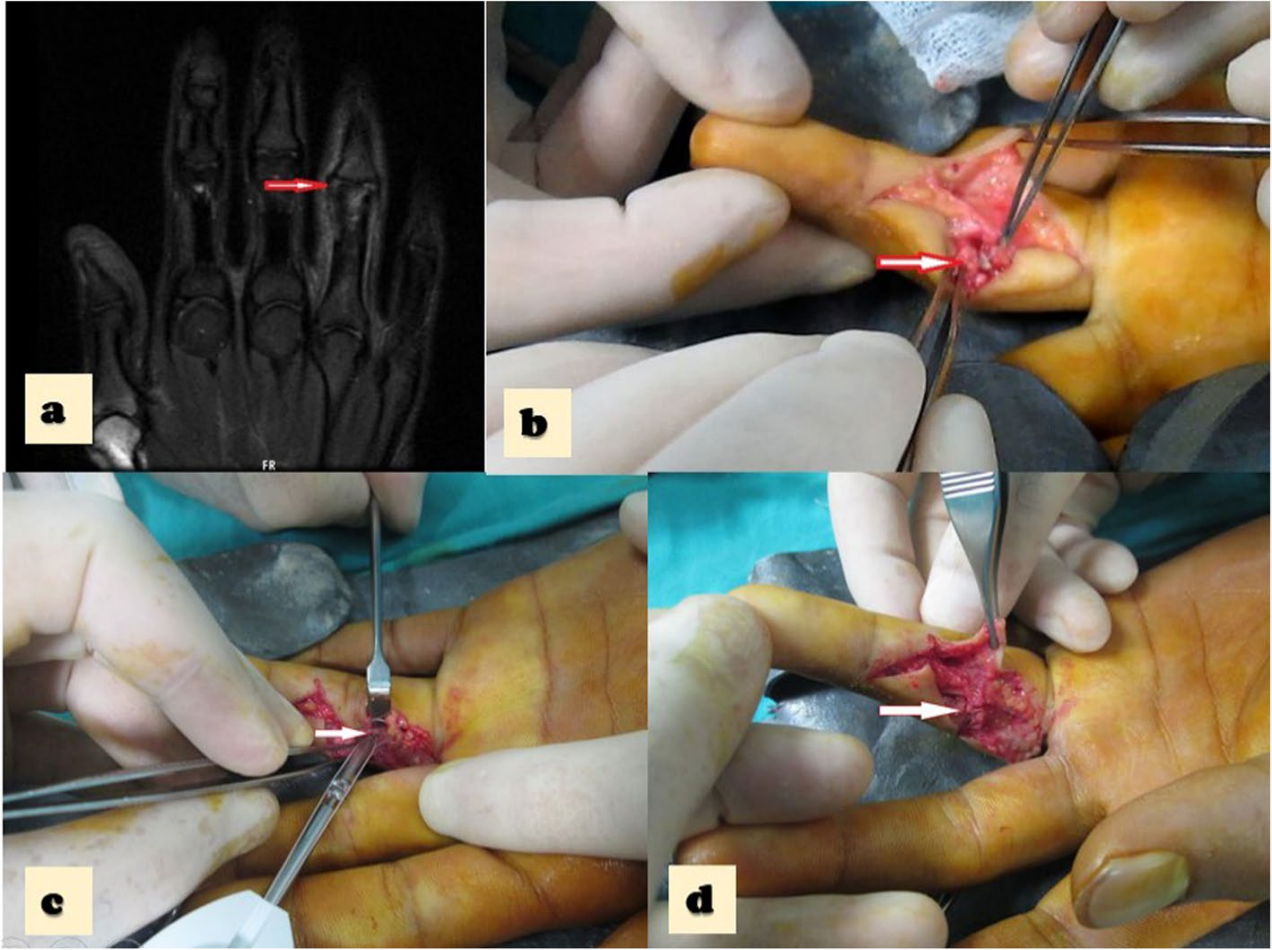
a The pre operative MRI that demonstrates the forth finger RCL rupture. b The intraoperative photo, notice the arrow that demonstrates the 4.^th^ finger RCL rupture. c The intraoperative photo notice the white arrow that demostrates the suture anchore augmented repair. d The intraoperative photo notice the white arrow that demostrates the completed repair side
